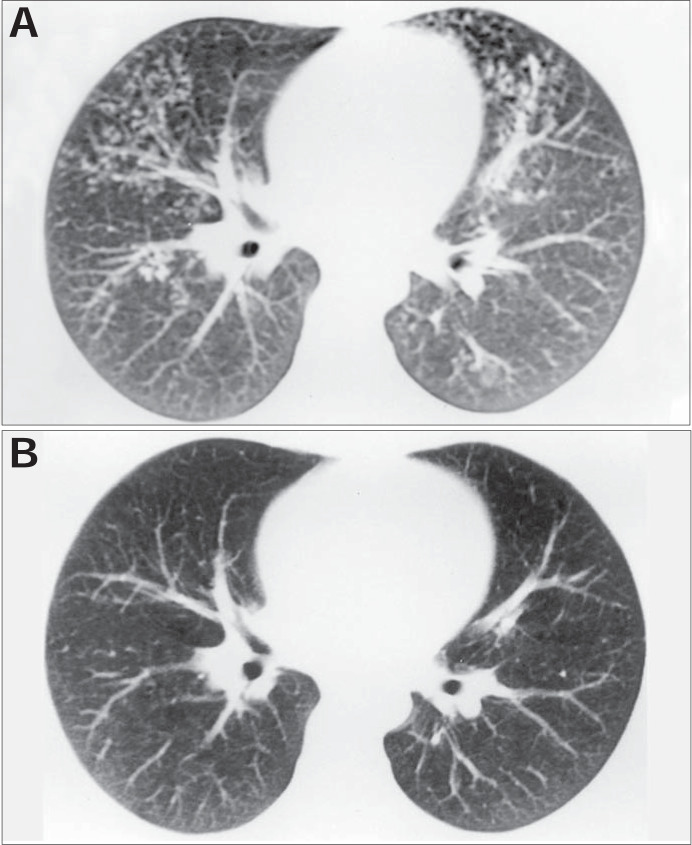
(A) Computed tomography at the start of treatment: centrolobular nodules with segmental distribution in the anterior parts of both lungs. (B) Computed tomography post-treatment, for the same patient: absence of the alterations observed at the start of treatment.
